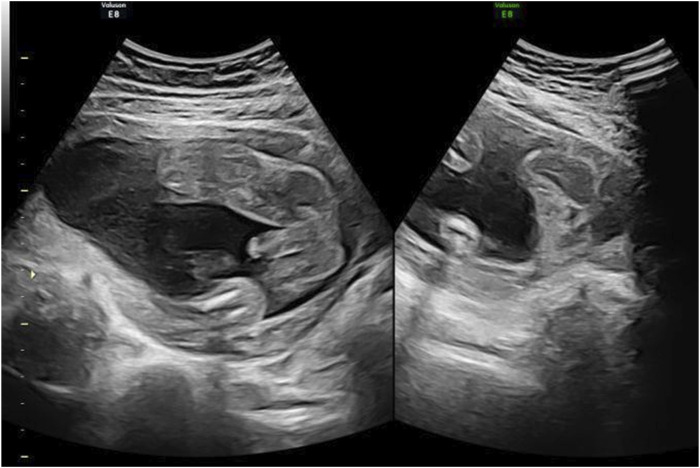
4D ultrasound of genital development at 24 weeks in the spironolactone-exposed pregnancy. The ultrasound shows no obvious abnormalities in the fetus.
